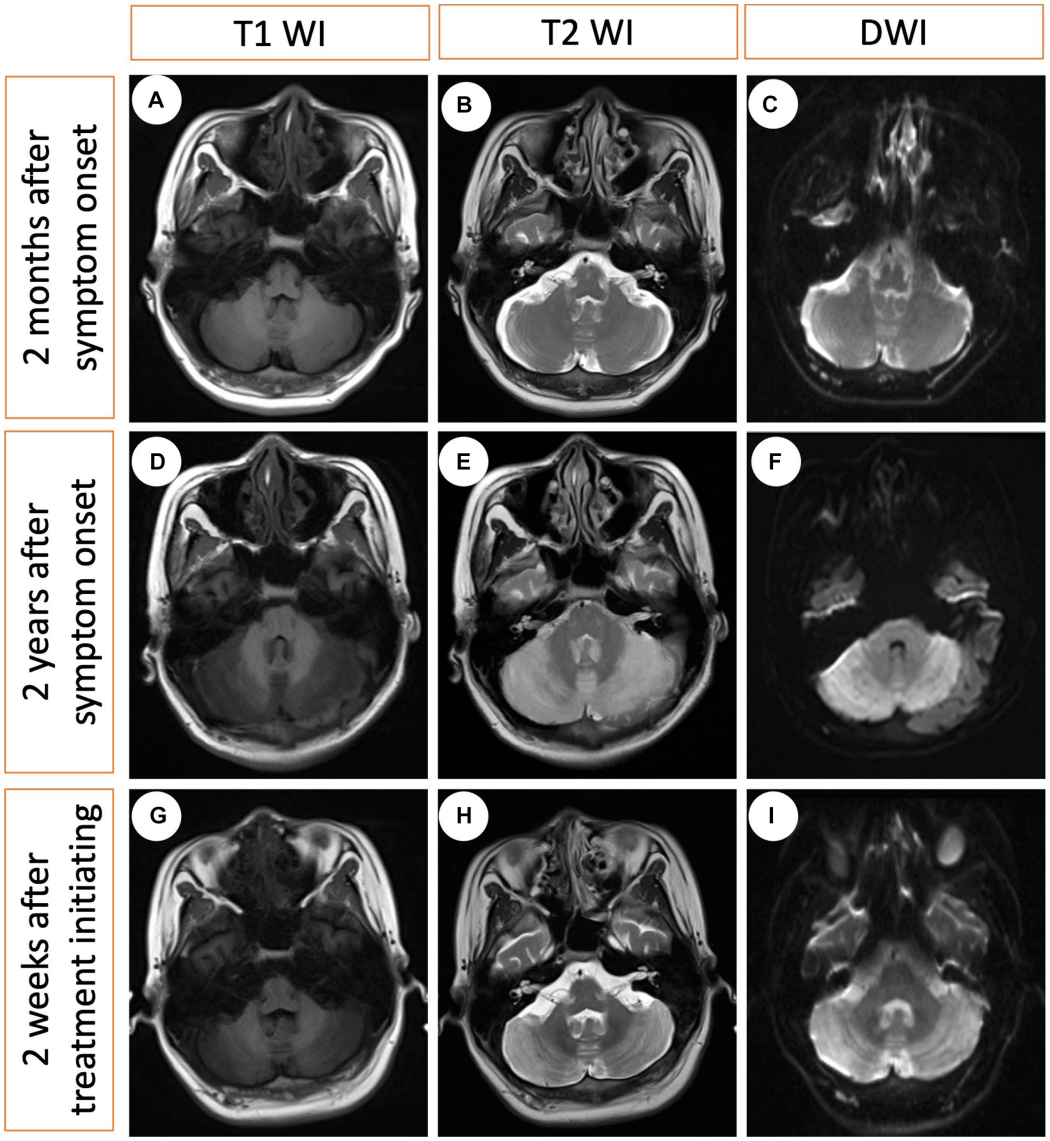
Serial MRI changes at three different time points. (A–C) The first MRI obtained 2 months after symptom onset (no treatment), indicating modest cerebral atrophy. (D–F) The second MRI obtained 2 years after symptom onset (no treatment), revealing bilateral hyperintensity in the cerebellum on the T2-weighted image and diffusion-weighted imaging (DWI), with hypointensity on the T1-weighted image. (G–I) The third MRI obtained 2 weeks after treatment initiation indicating minimal changes.
